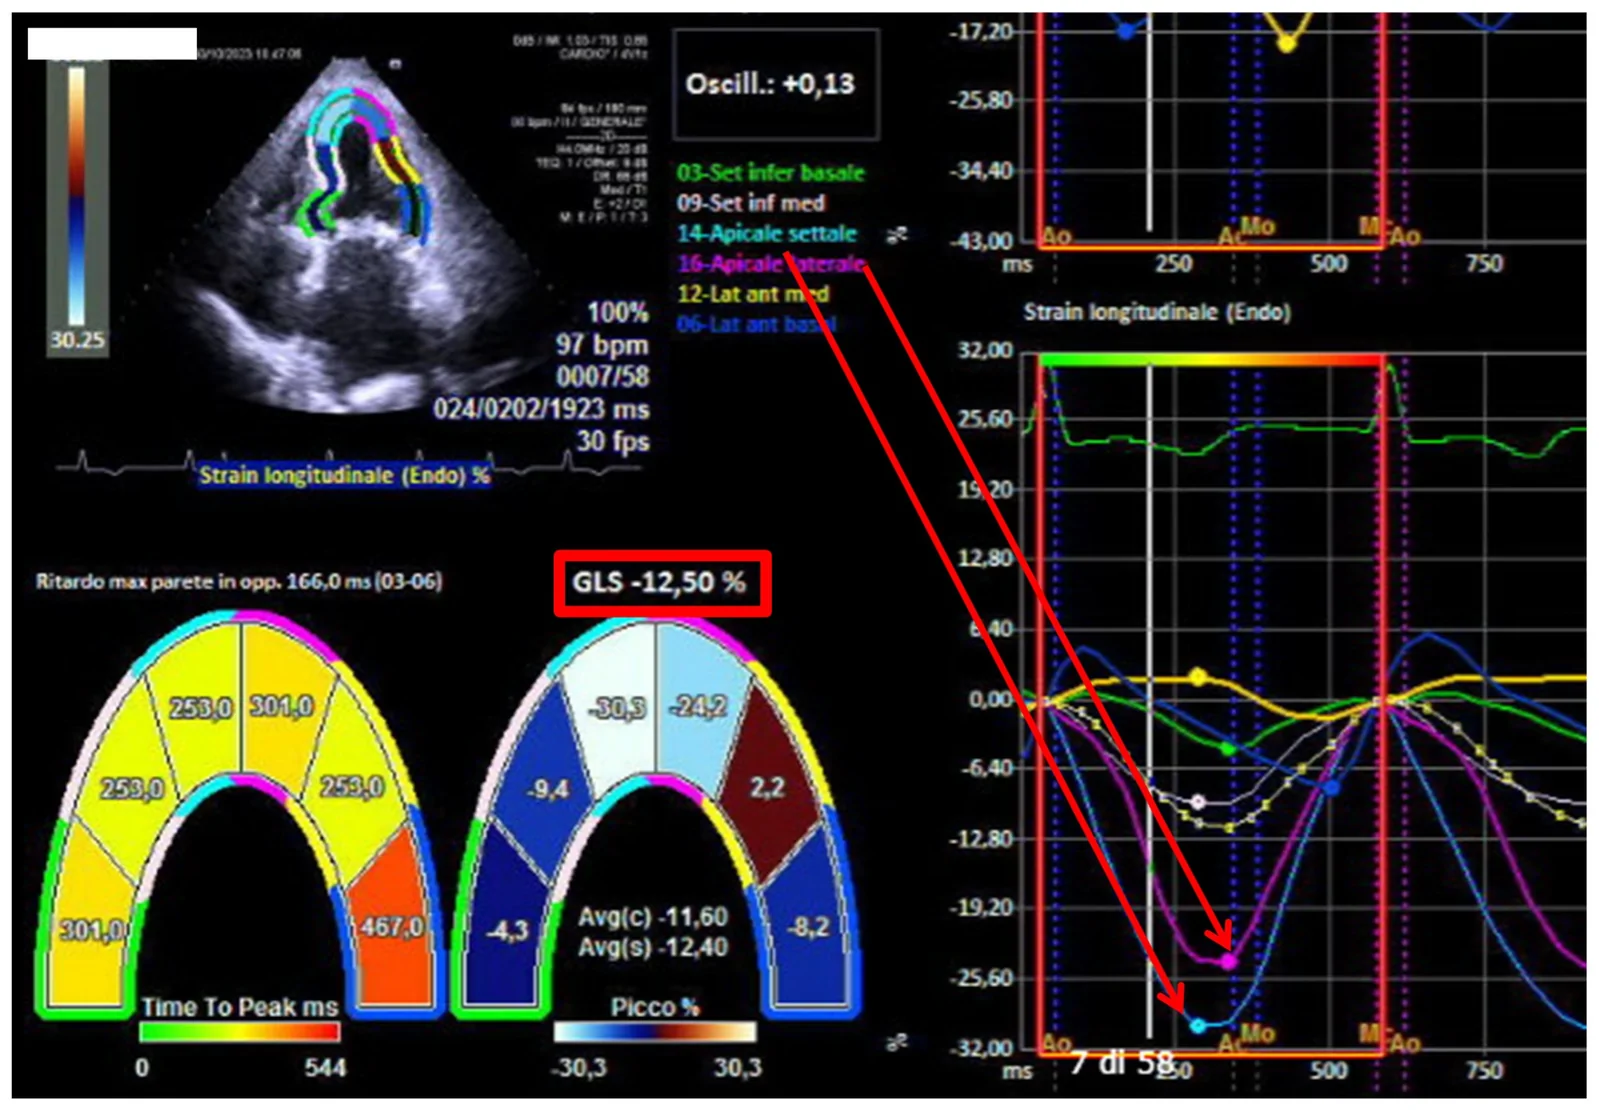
Speckle-tracking echocardiography; 4-chamber view. Impaired global longitudinal strain (−12.5%) with preserved deformation of septal and lateral apical segments (arrow), depicting the typical “apical sparing” pattern in a patient with TTR cardiac amyloidosis.
